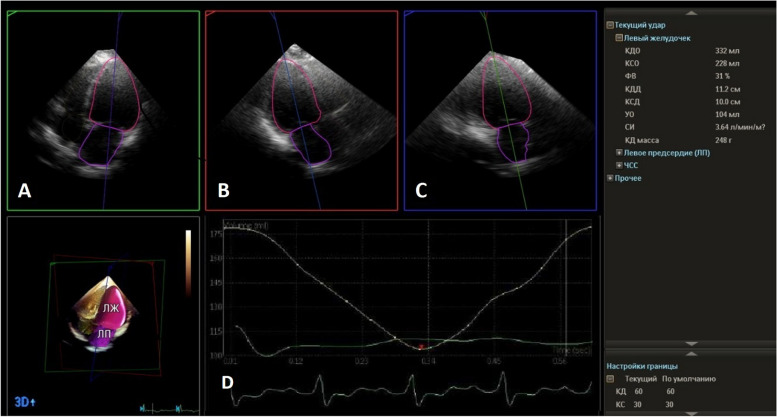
Semi-automated 3D echocardiography. The 3D data set can be analyzed and sliced and 4-chamber (Panel A), long-axis (Panel B), and 2-chamber views (Panel C) can be obtained. Time/volume curves can be automatically displayed (Panel D) which provide measurements of LV volumes and ejection fraction
